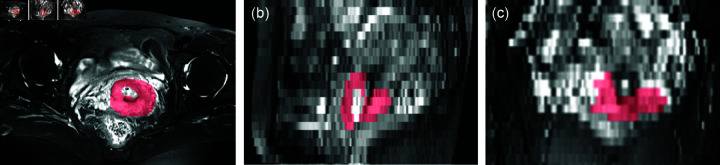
Three-view images showing the tumor location in T2-weighted MRI of a cervical cancer patient. (a) Axial image and label. (b) Sagittal image and label. (c) Coronal image and label.
